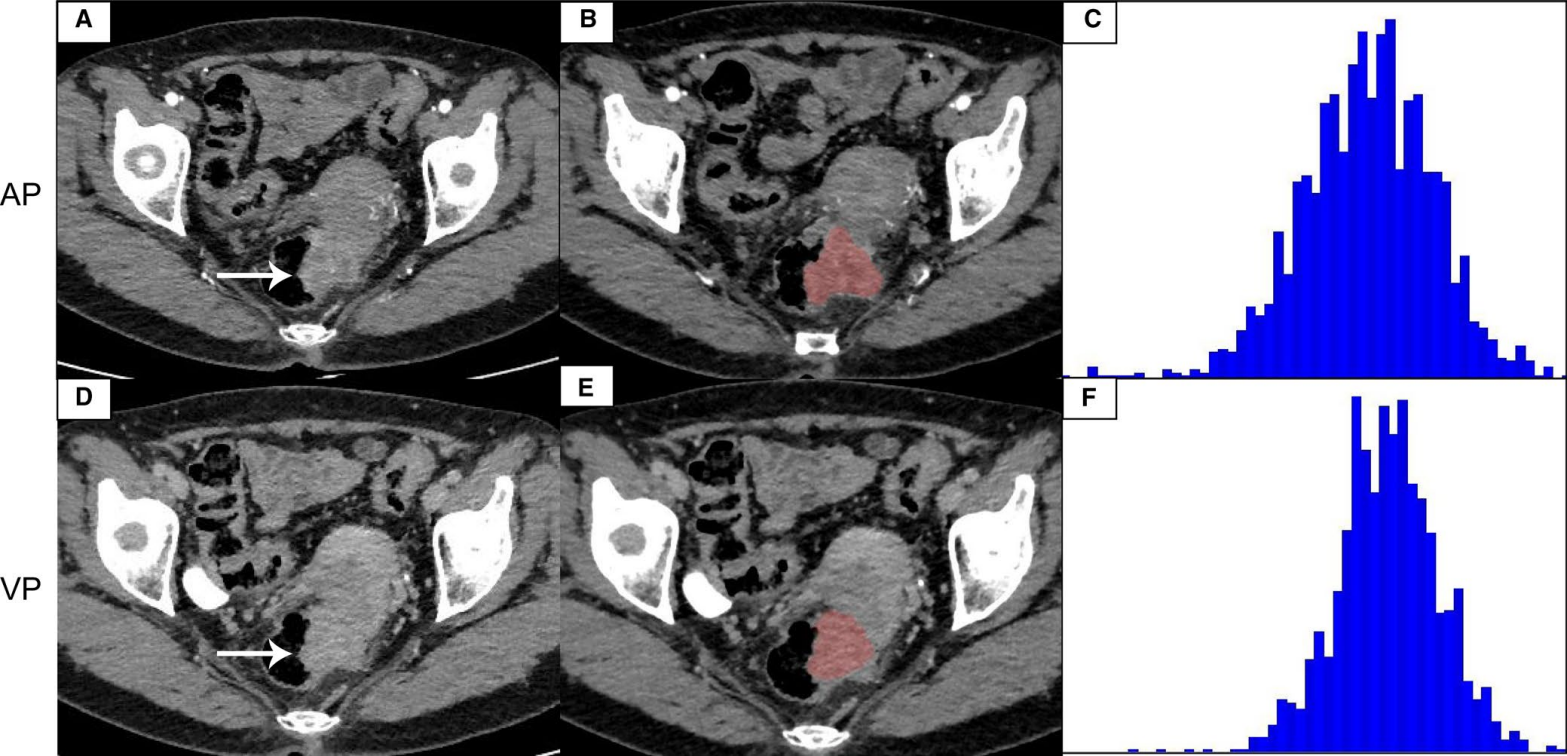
A 54‐year‐old female with G2 R‐NETs. The tumor was significantly enhanced on the arterial phase image (A), and the degree of enhancement is reduced on the venous phase image (D). The tumor region of interest (ROI) was localized on axial CT images at arterial phase (B) and venous phase (E). CT histogram at arterial phase (C) and venous phase (F) were shown.
